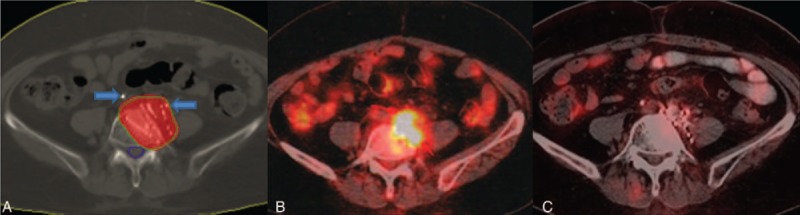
(A) The inner green curve represent the PTV. The blue curve represent spinal cord. The red area is covered by 90% prescribed dose (108 Gy). Arrows point ureteral stents. (B) PET-CT scan before ^125^I implantation treatment. (C) PET-CT scan at 5 months after treatment.
